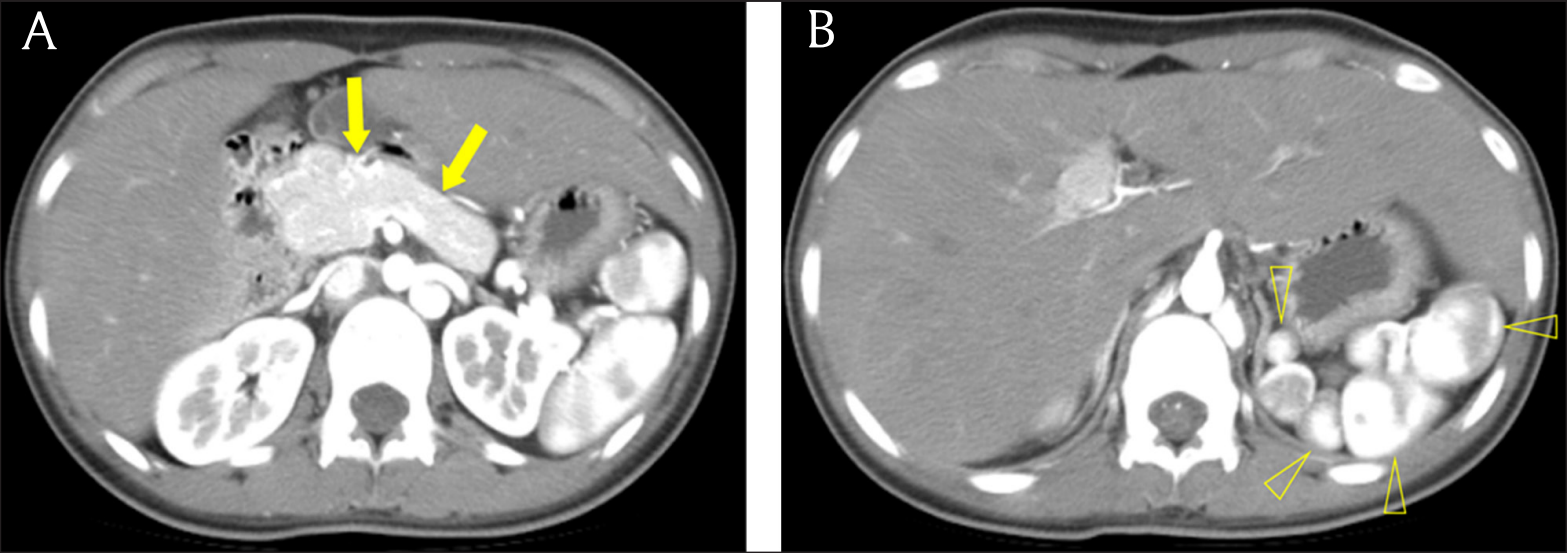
A 45-year-old man with heterotaxy syndrome. (A) Axial pancreatic phase CT image shows the short pancreas (arrows), suggesting partial agenesis of dorsal pancreas. (B) The multiple splenic tissues (open arrowheads) are located in the left side of the abdomen, indicating polysplenia.
